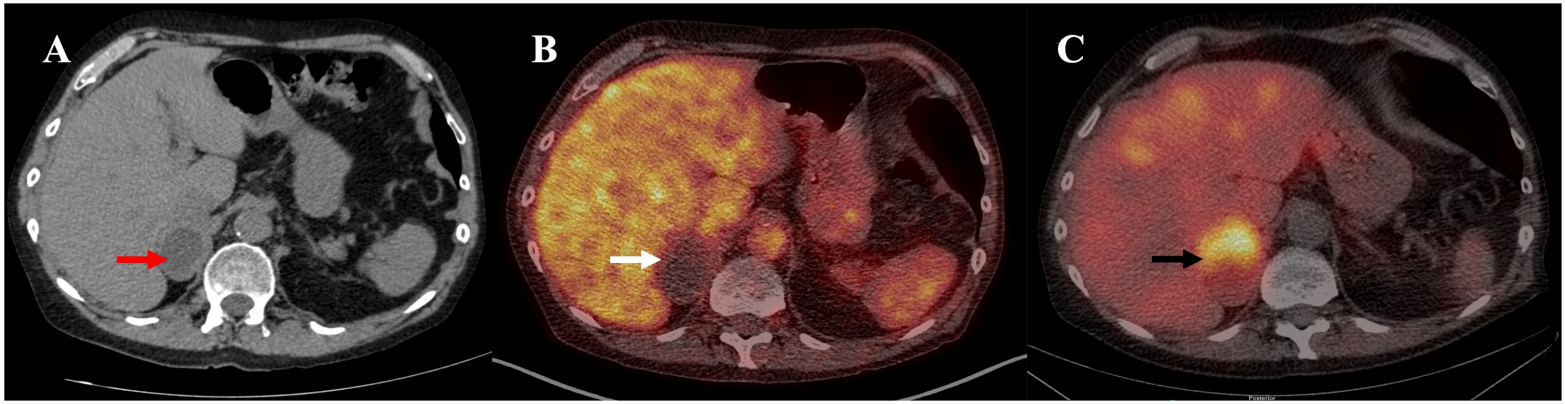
(A) Unenhanced CT: heterogenous 5.8 cm x 3.9 cm right adrenal mass with a density of 7 Hounsfield Units (HU) (red arrow). (B)
